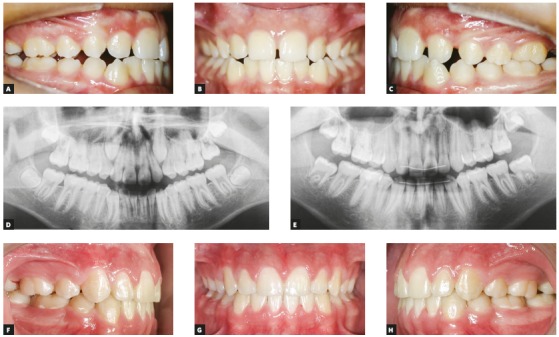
A-D) Pretreatment intraoral photographs and panoramic radiograph. E-H) Postreatment intraoral photographs and panoramic radiographs. Teeth #14, #24, #35 and #45 were extracted.
